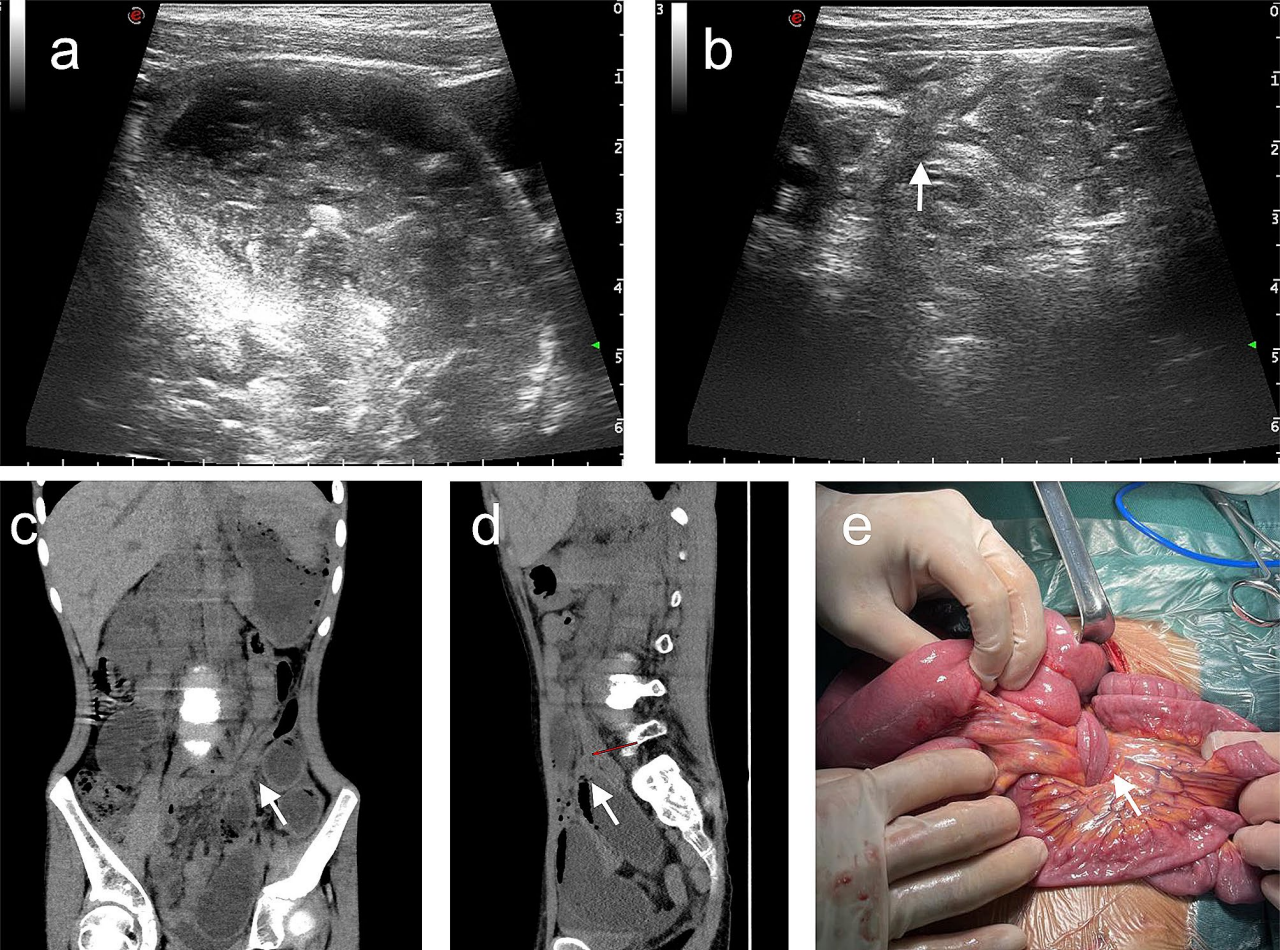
Representative imaging scans of a 7-year-old male patient presenting abdominal pain. A, B: Ultrasound scans visualizing an IAH complicated with intestinal dilation and obstruction, thickening of the intestinal wall, liquid exudation in the surrounding intestinal space, and a “cross sign” ( white arrow) of the adhesive bands in the dilated intestinal tubes. C, D: Coronal and sagittal CT scans of the abdomen showed dilatation of intestinal cavity in the left middle and lower abdomen, intestinal effusion, thickening of intestinal wall and visible gas accumulation. The obstruction point (white arrow) was visualized, where the intestinal tube was entangled with the hyperechoic mesentery. E: Intraoperative manifestations of an IAH, including the banding between the beginning of jejunum and the ileum, twisted proximal intestine, extensive adhesion between the small intestine, and a C ring at the adhesive bands (white arrow)
